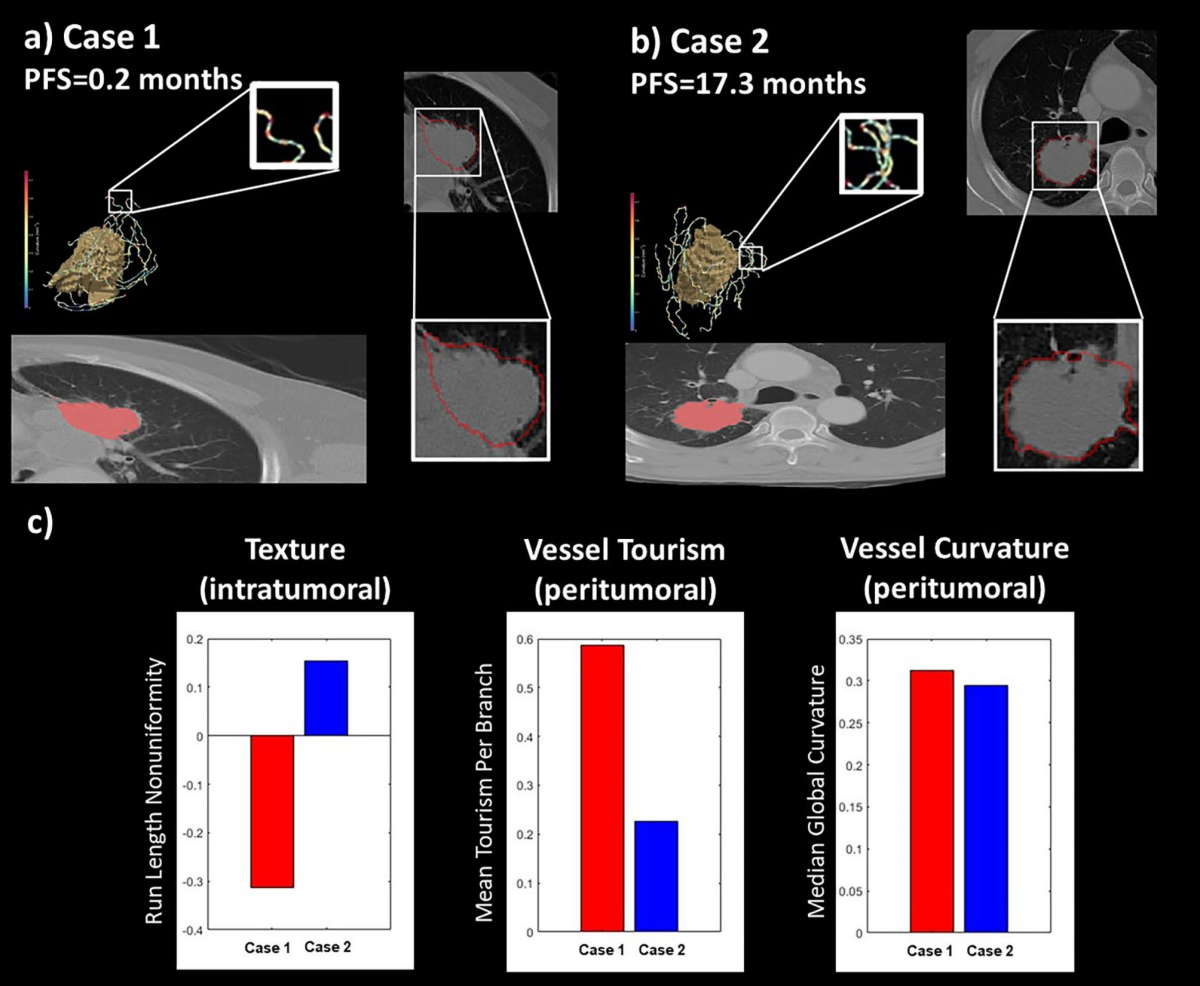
Representative cases for the IO outcome prediction. The outcome of IO treatment is associated with the characteristics of the peritumoral vasculature and the physical properties of the tumor as observed on CT images. In two cases with similar tumor size, Case 1 (a), with a poorer prognosis, exhibited higher local heterogeneity of the tumor and curvature and tortuosity of the peritumoral vessels compared to Case 2 (b), with a better prognosis. Case 1 (a) also showed growth primarily in the area near the chest wall, with limited distribution of blood vessels around the tumor. These findings suggest that the effects of IO treatment and drug delivery may be influenced by these factors
